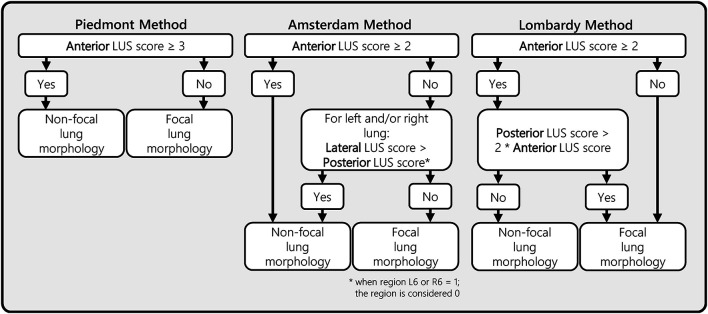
Ultrasound lung morphology assessment methods. This figure presents three LUS morphology assessment methods that were designed and/or evaluated in this study. All three methods classify focal or non-focal lung morphology based on LUS aeration scores from a 12-region LUS exam. The anterior, lateral, and posterior LUS scores were defined as the sum of the LUS aeration score in the four anterior, lateral, and posterior regions, respectively. The Piedmont method was previously proposed in a study from Costamagna et al. (2021). The Amsterdam and Lombardy method were developed for the purpose of this study by two expert panels from the corresponding regions. LUS, lung ultrasound.
